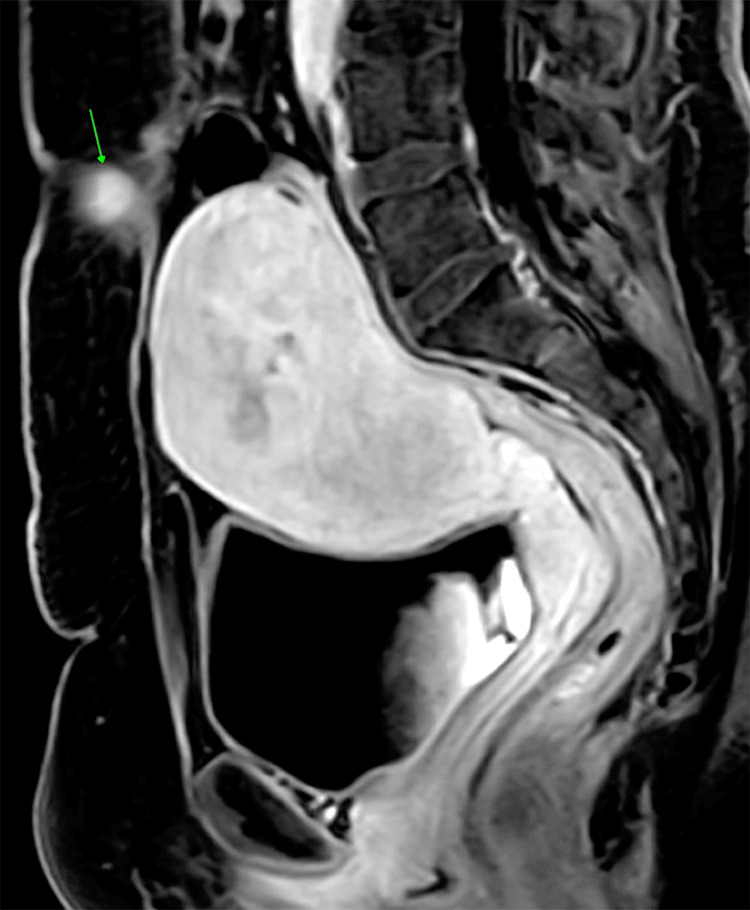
Sagittal T1 FS postcontrast MRI sequence shows enhancing subcutaneous solid lesion (arrow) within the umbilicus. No intraperitoneal extension is seen. Anteverted bulky uterus is seen due to an intramural leiomyoma
